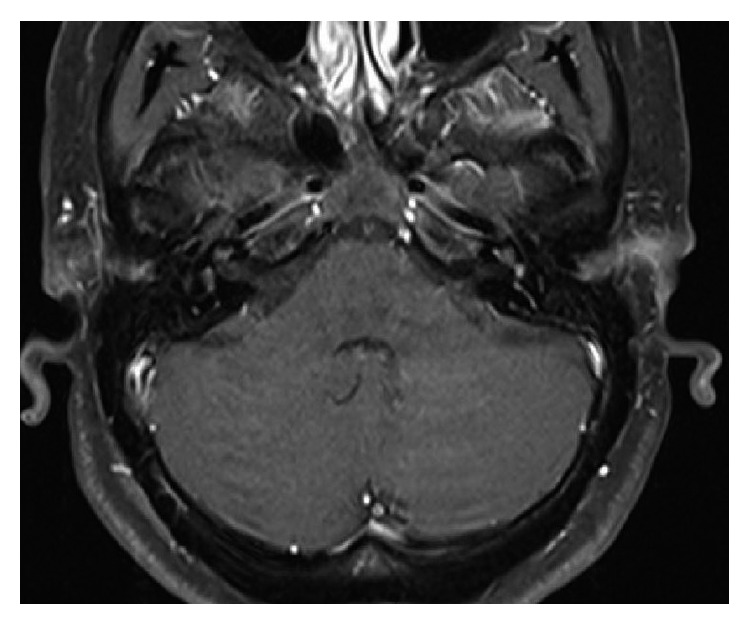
MRI T1 axial postcontrast imaging demonstrating bilateral enhancement of the cochlea, vestibule, and semicircular canals.
